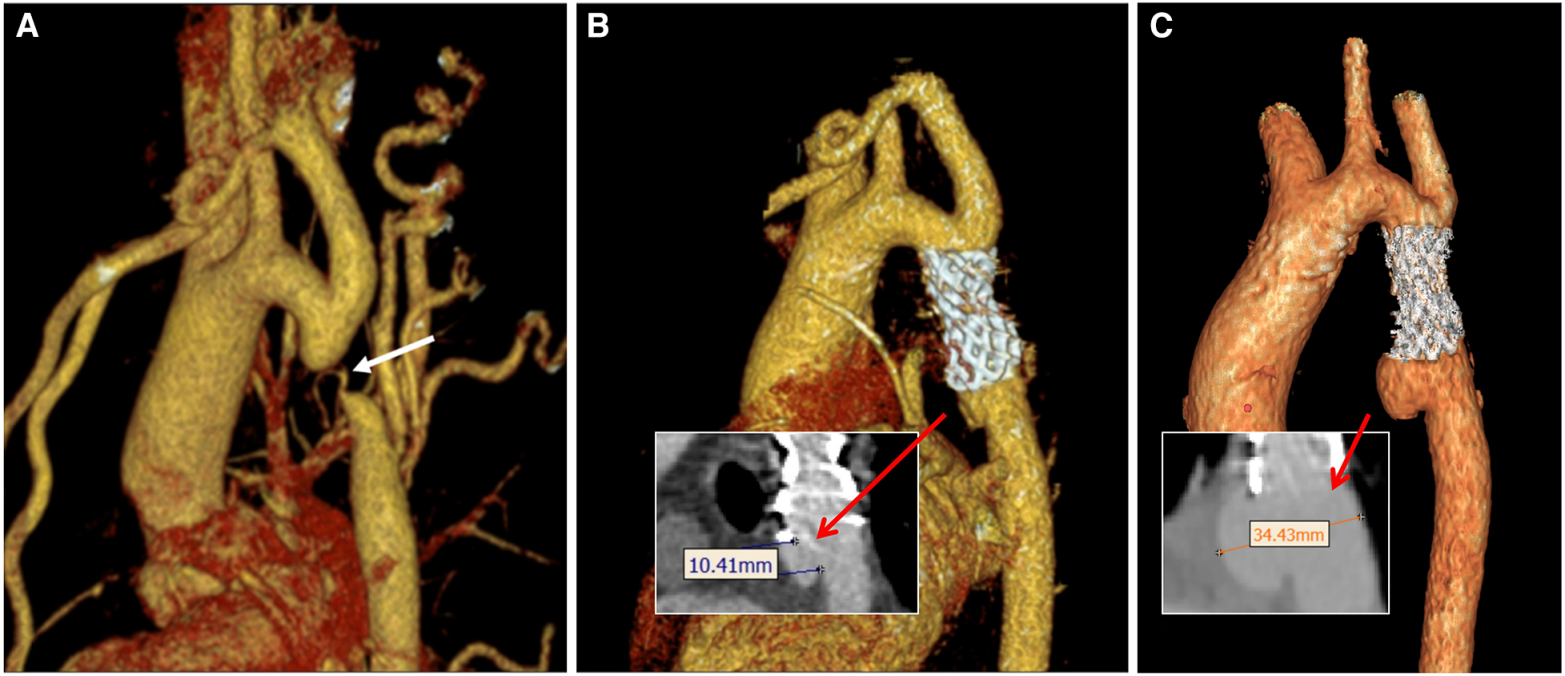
Three-dimensional images of the aorta during perioperative CTA in patient. (A) Preoperative image; (B) postoperative-3 days; (C) postoperative-18 months. The white arrow indicates the position of the stenosis. The red arrows indicate the neck of the aneurysm in B and the distance between the aneurysm dome and the parent artery in C, respectively.
